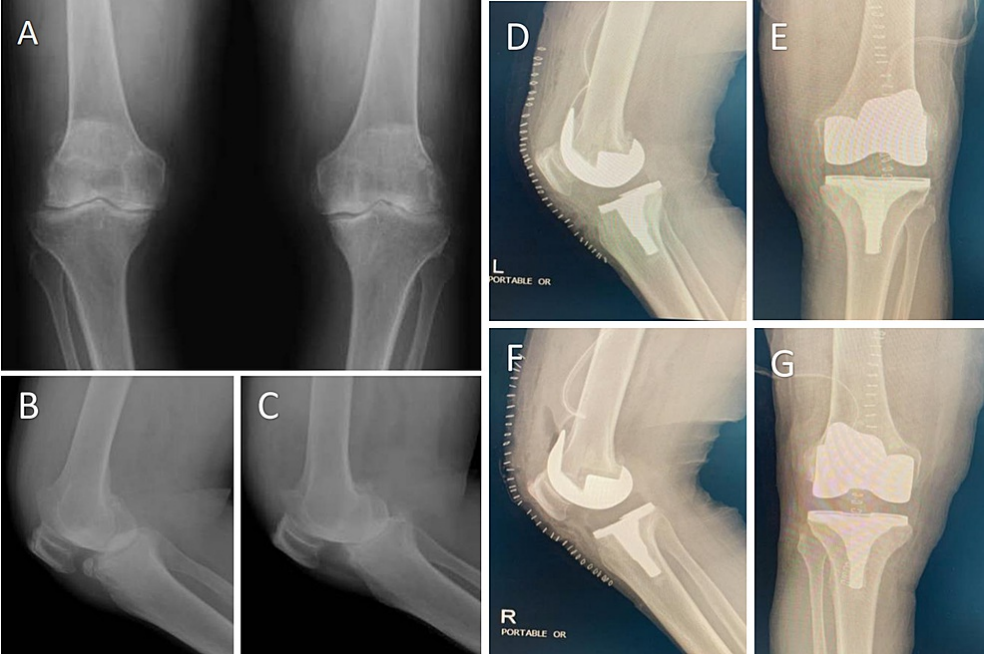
Pre-operative X-ray and post bilateral total knee arthroplasty X-ray Pre-operative X-ray shows tri-compartments advanced osteoarthritic changes at both knees manifested by significant joint space loss, subchondral sclerosis, and marginal osteophytes formation. Bilateral moderate effusions are seen. Mild genu varus deformity was noted on both sides (A-C). Post bilateral total knee arthroplasty X-ray study shows intact hardware appearance and anatomic alignment (D-G).
